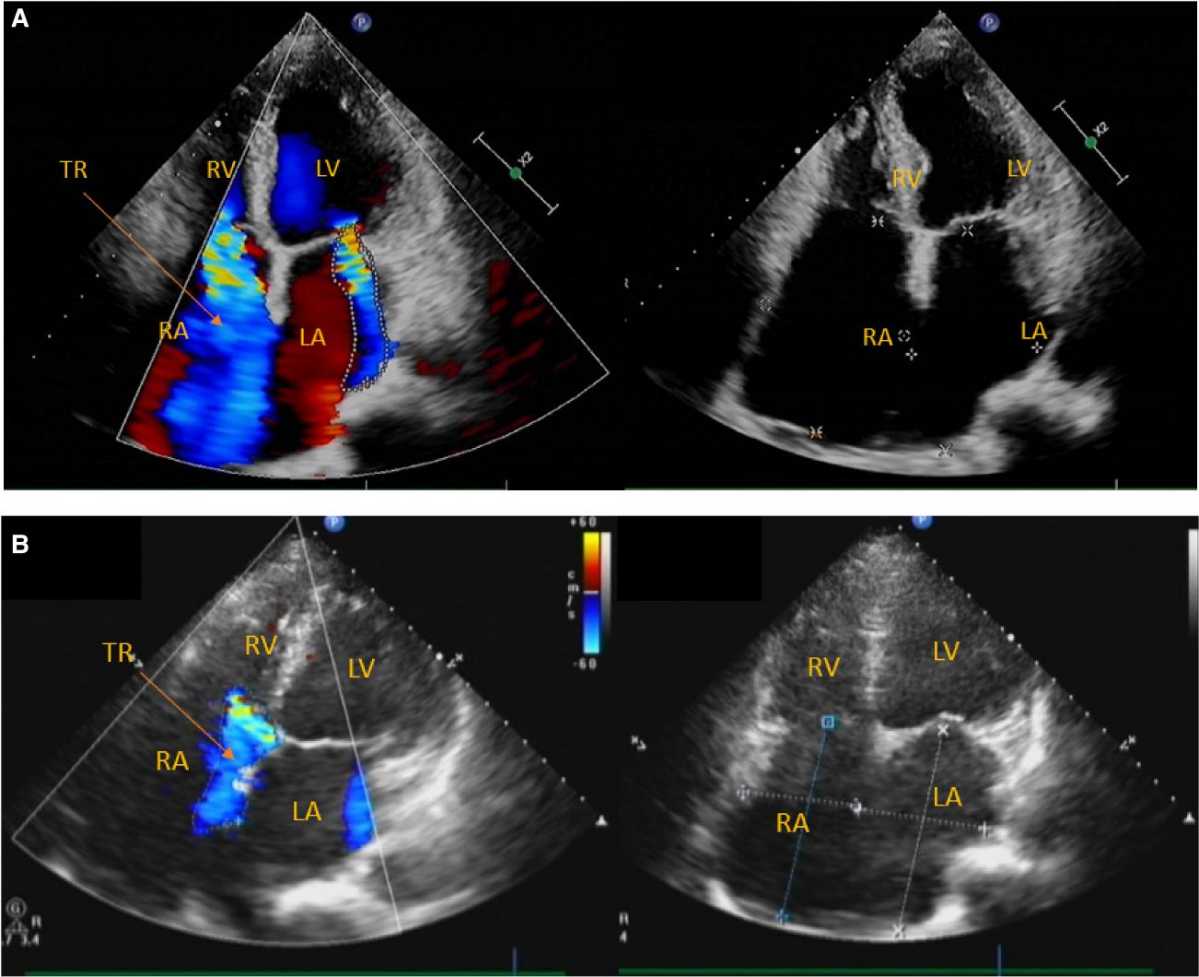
(*A*) Echocardiography showing large lead-related tricuspid regurgitation (pointed by the arrow) before lead extraction (ejection fraction: 50%, the end-diastolic dimension of the left ventricle: 53 mm, the area of tricuspid regurgitation: 20 cm^2^, Vmax of the tricuspid regurgitation: 3 m/s, pressure gradient across tricuspid valves: 35 mmHg; right atria size: 51.3 cm^2^, the left atria size: 24 cm^2^). (*B*) Echocardiography showing significantly reduced tricuspid regurgitation (like the arrow pointing) and decreased atrial size after the lead extraction (EF: 62%, LVED: 57 mm, the area of tricuspid regurgitation: 8.1 cm^2^, Vmax of the tricuspid regurgitation: 3 m/s, pressure gradient across tricuspid valves: 35 mmHg; the right atria size: 39 cm^2^, the left atria size: 22.8 cm^2^).
